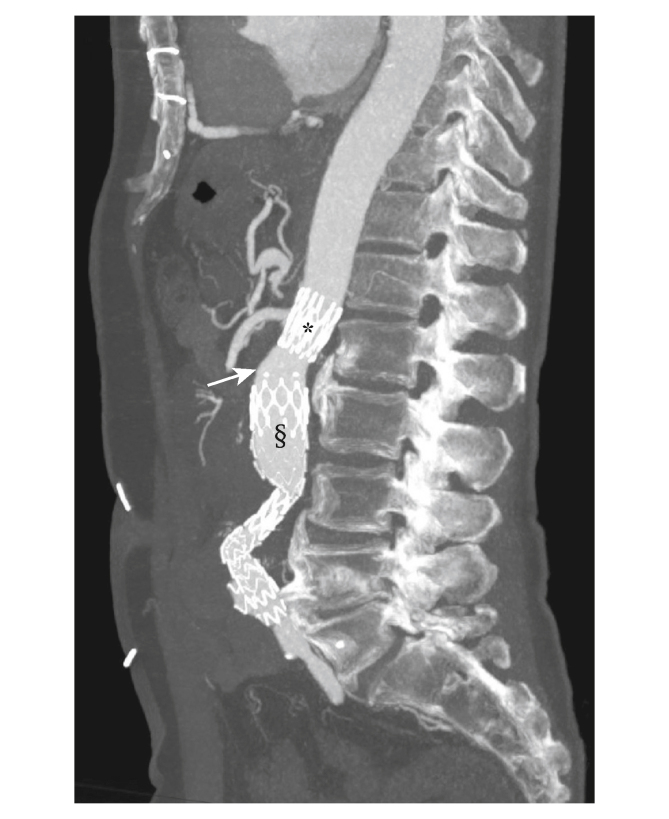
Computed tomography angiogram (sagittal plane) of a 78 year old male patient with abdominal aortic aneurysm showing separation (white arrow) of the suprarenal bare metal stent (∗) from the graft main body (§) after endovascular aneurysm repair.
